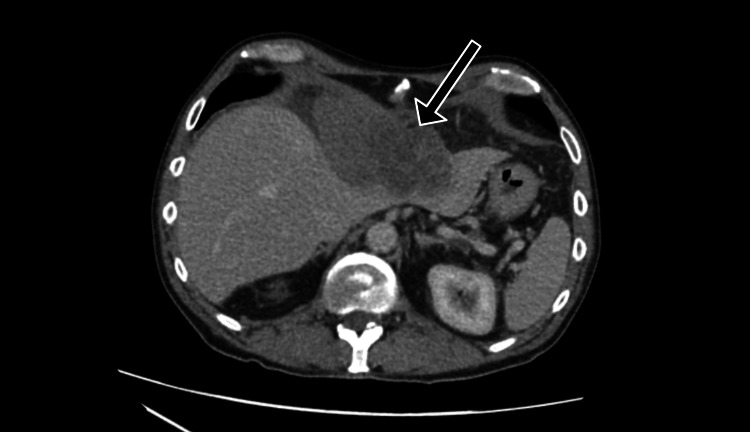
Axial portal venous phase CT of the abdomen showing a large epigastric mass with evidence of local invasion into the liver.
